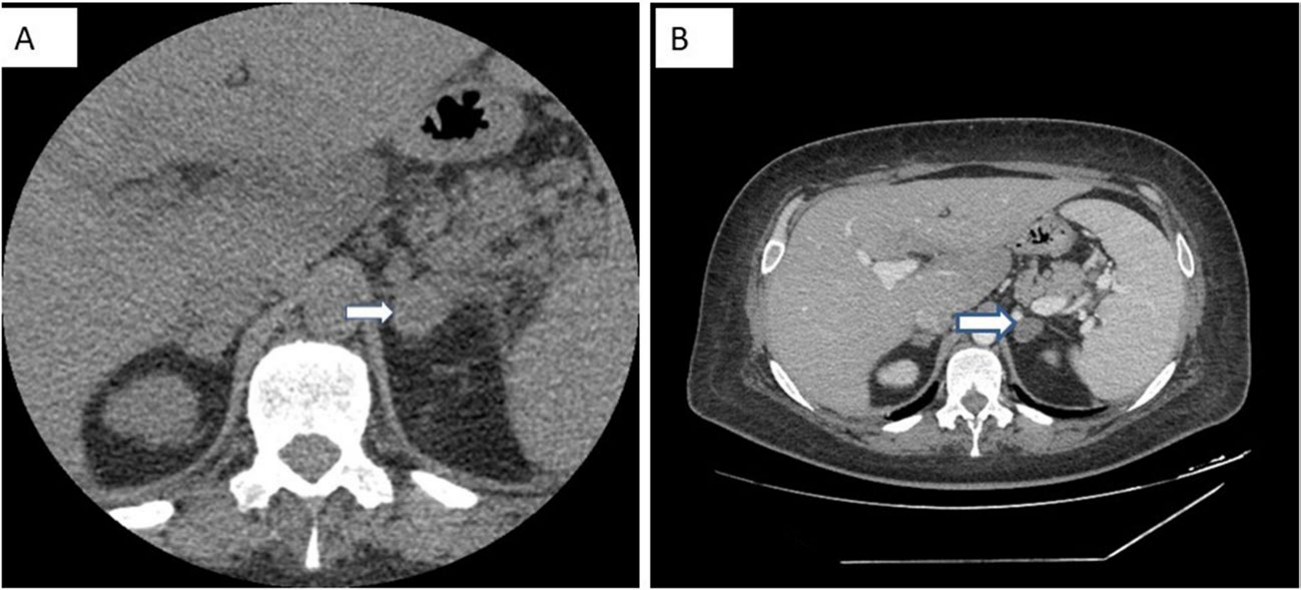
A Adrenal computed tomography (CT) showing bilateral enlargement of the adrenal glands mainly of the left, with intermediate mean density, punctate calcifications, and perinephric fluid, consistent with a recent bilateral adrenal hemorrhage. B Repeat adrenal CT scan showing a decrease in size of both adrenal glands, suggesting remission of the hemorrhage
